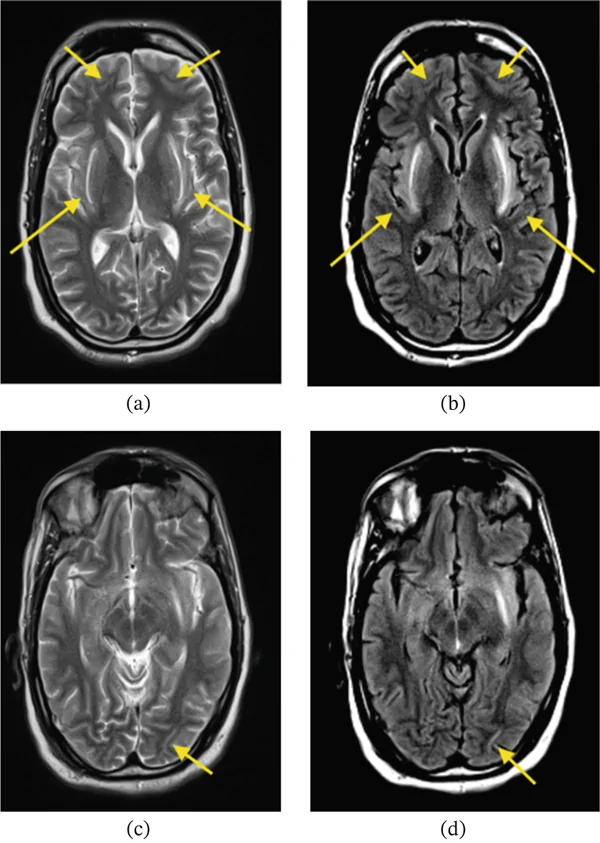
Brain MRI hospital Day 10. (a) T2‐ and (b) FLAIR‐weighted MRI scans at the level of basal ganglia (axial view) showed bilateral putamen, external capsule, and anterior frontal subcortical white matter with abnormal T2/FLAIR hyper‐intensity (yellow arrows). (c) T2‐ and (d) FLAIR‐weighted MRI scans at the level of occipital lobes (axial view) showed left occipital subcortical white matter with abnormal T2/FLAIR hyper‐intensity (yellow arrows). Teaching point: T2/FLAIR sequences demonstrate characteristic bilateral putaminal injury with additional frontal and occipital subcortical white matter involvement.
